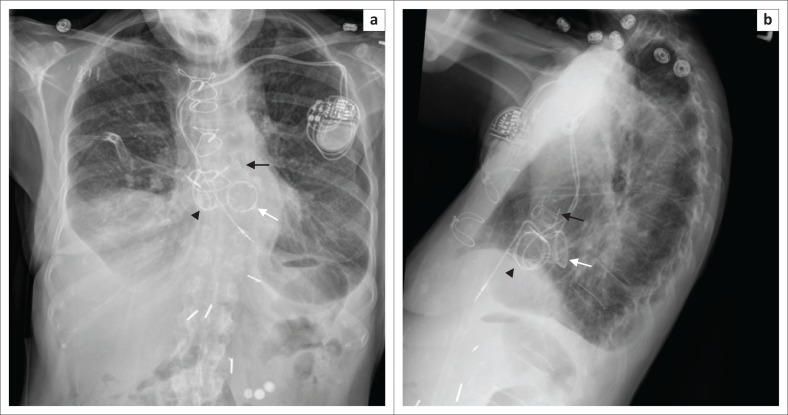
Frontal (a) and lateral (b) chest radiographs showing prosthetic aortic (black arrow), mitral (white arrow) and tricuspid (arrow head) valves in a 72-year-old female patient with a history of aortic and mitral stenosis, tricuspid regurgitation and complete heart block.
